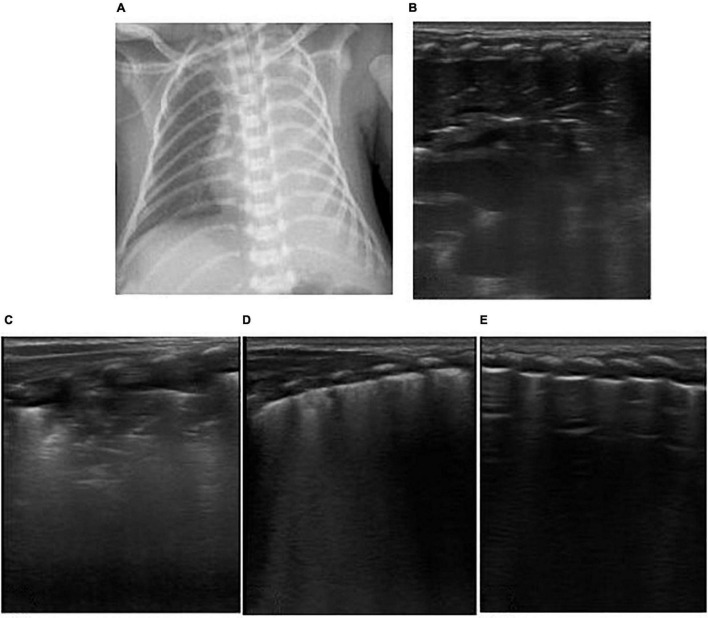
The patient was born at 33^+1^ weeks of gestational age by Cesarean section due to the mother’s placenta previa and hemorrhagic shock, with an inborn weight of 1.75 kg and hemorrhagic amniotic fluid. The Apgar score of 1, 5, and 10 min of birth were 1, 7, and 7 points. The patient had pulmonary hemorrhage after delivery. Ventilator weaning was performed after 10 days of mechanical ventilation. After 2 days post-ventilator weaning, the patient had decreased blood oxygen and labored breathing. Mechanical ventilation was reperformed *via* endotracheal intubation. Emergency LUS showed that the left lung showed liver like echo, pleural line, and A line disappeared, dendritic bronchial inflation sign and pulmonary pulsation were visible, revealed left lung atelectasis (B). Chest X-ray showed white lung changes in the left lung (A). Lung recruitment was performed under ultrasound monitoring, and the range of consolidation was reduced gradually, and pleural line and A-lines were clear (C–E). Lung ventilation improved, and finally, the ventilator was weaned.
